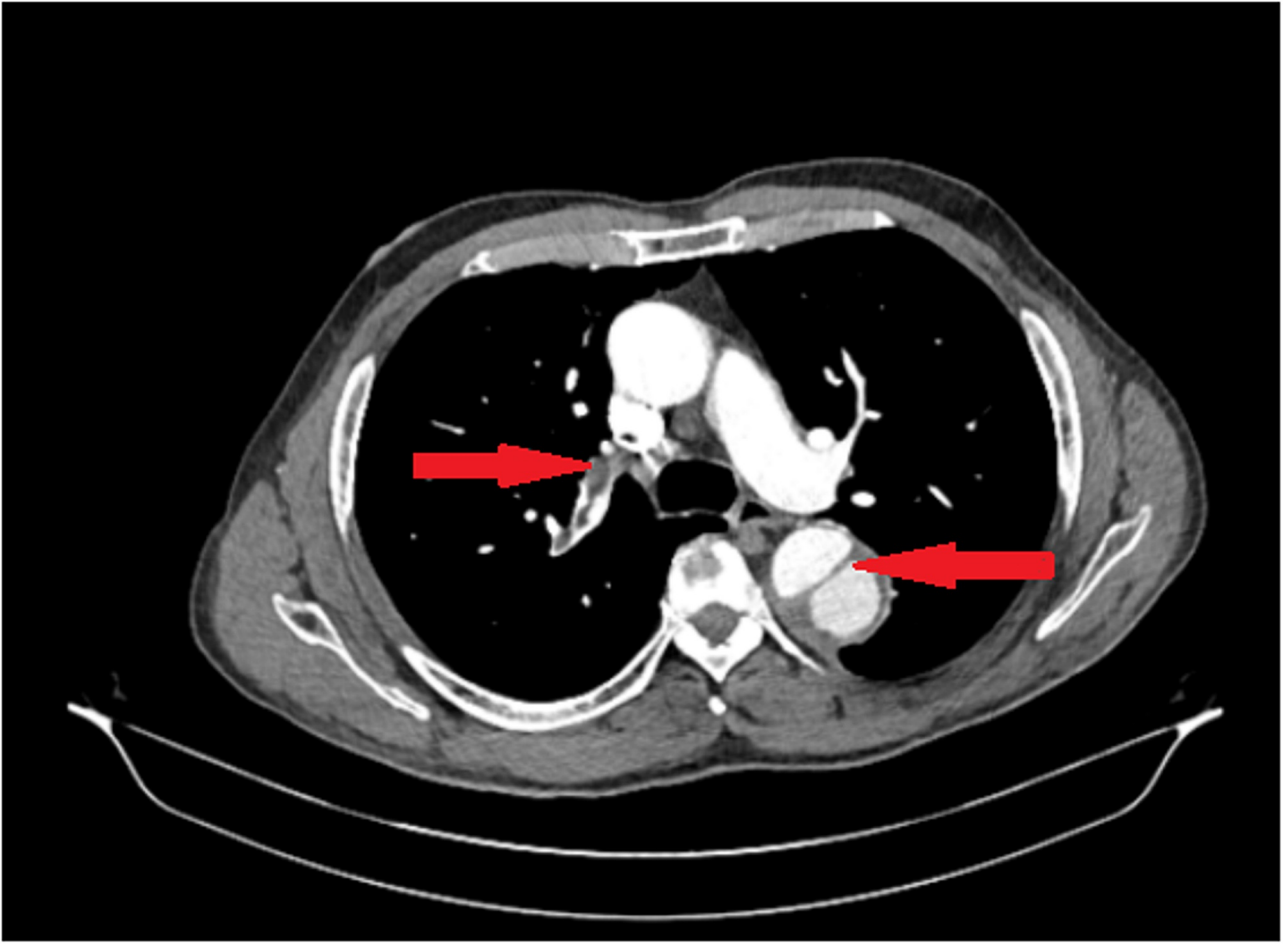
CT angiography showing embolus and aortic dissection in descending aorta
